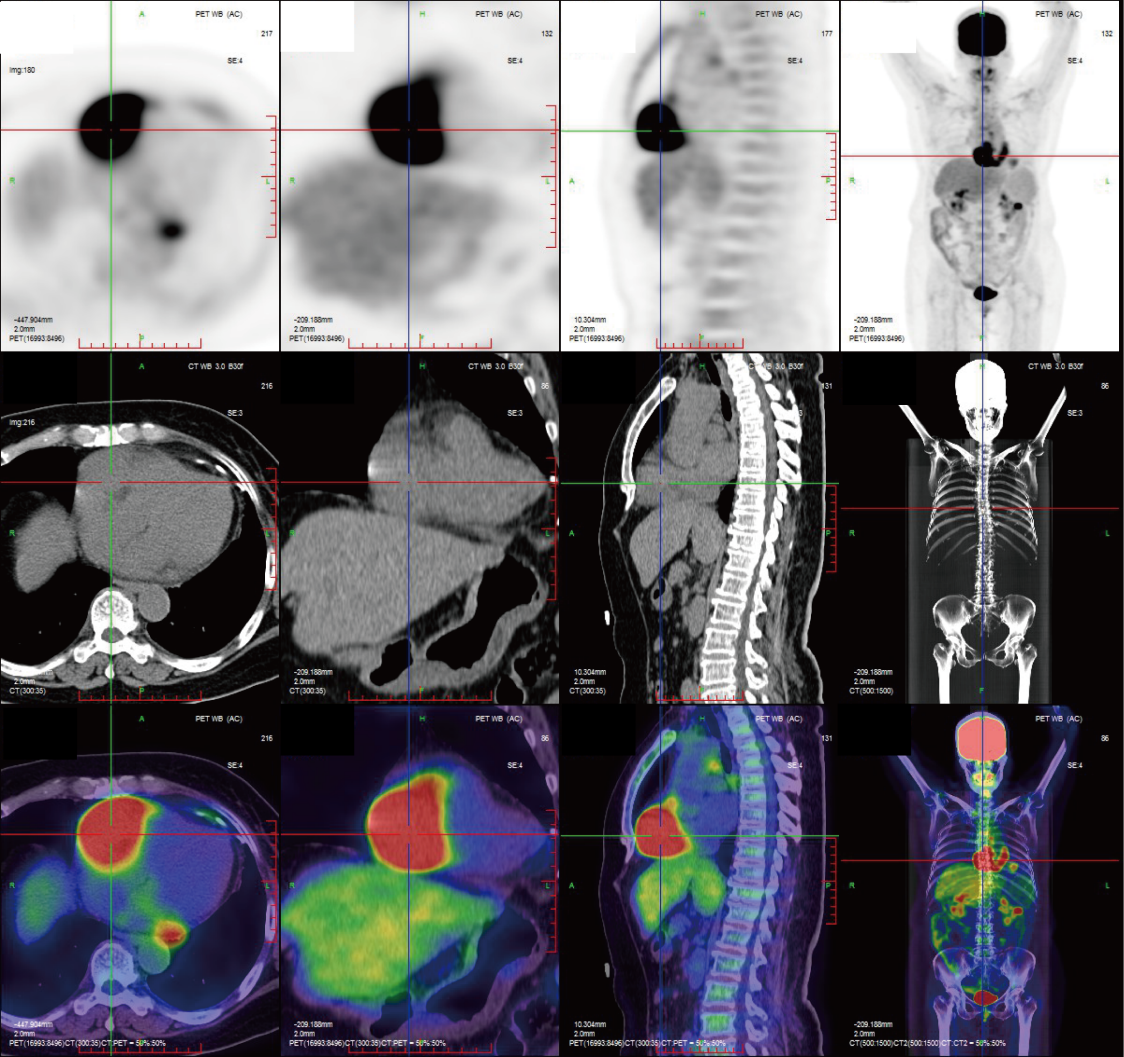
Preoperative PET/CT imaging. PET-CT imaging identified an irregular, iso-dense soft tissue mass in the right atrioventricular region extending toward the left ventricular margin, with markedly increased metabolic activity, highly suggestive of a malignant process. No distant metastatic foci were detected.
